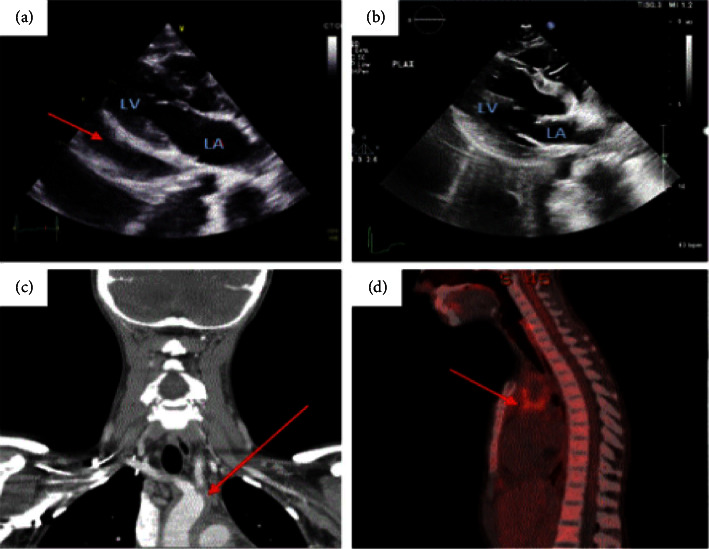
Diagnostic imaging of a patient with Takayasu arteritis. (a) Initial echocardiogram with a large pericardial effusion, red arrow. (b) Repeat echocardiogram without pericardial effusion, obtained one week after starting steroids. (c) CT image of the neck in a coronal plane with thickened proximal aortic and great vessel walls prior to diagnosis, red arrow. (d) PET-CT image in a sagittal plane with active inflammation of the proximal aorta and great vessels, consistent with TAK, red arrow.
